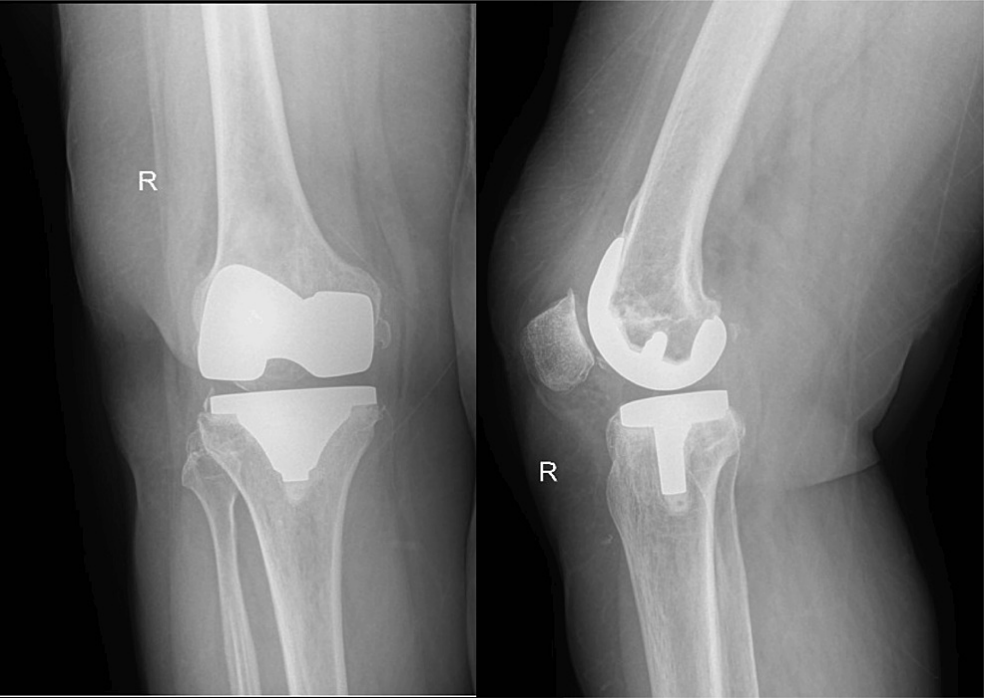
The anteroposterior and lateral views of the knee in the standing position These radiographs show a well-balanced knee; however, the patella position couldn't be commented on in these radiographs.
